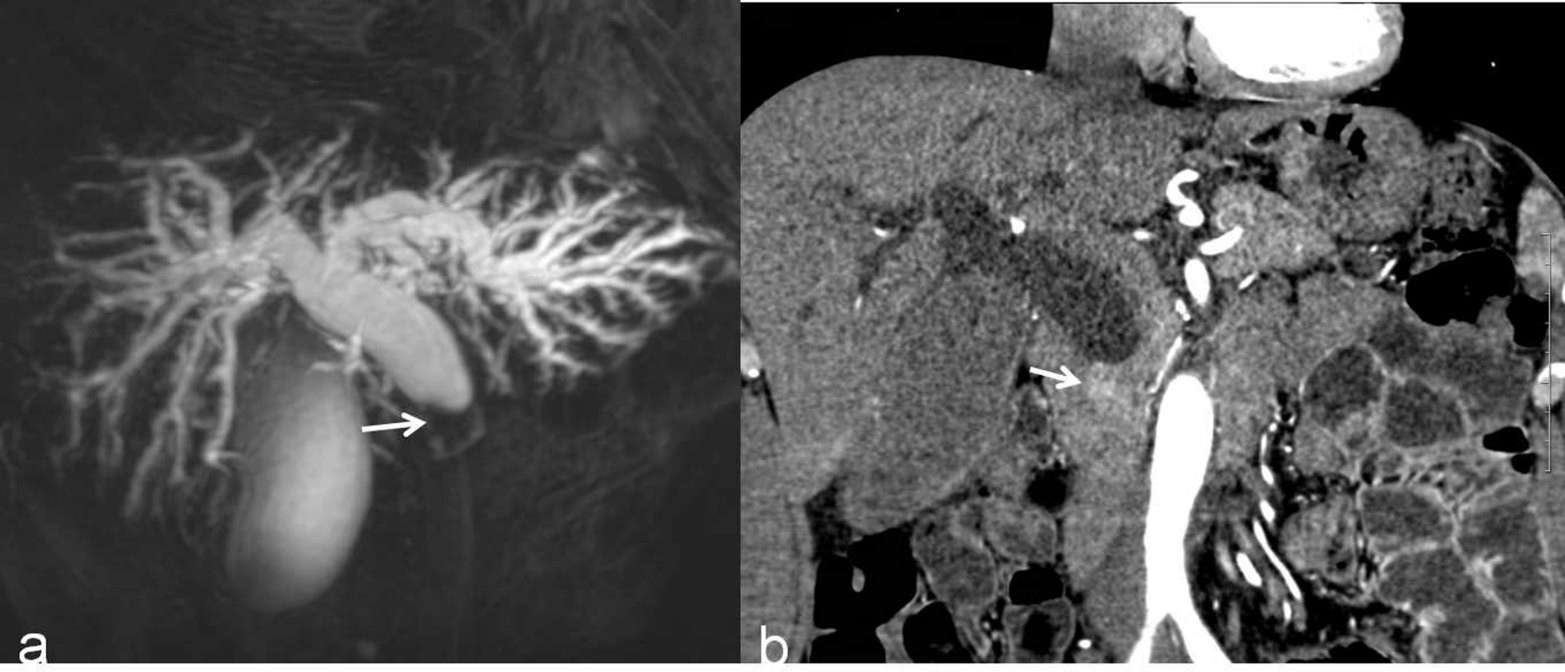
A malignant DBS (score=2) in a 59-year-old man confirmed the lesion as cholangiocarcinoma after surgery. (A) A 3D MRCP image shows abrupt, asymmetrical occlusion with irregular margins (white arrow). The length of the longest segment is 16 mm, the angle of the DBS is 100.4°, and the double duct sign is not present. (B) A coronal CT MPR image in the arterial phase shows the thickened wall with hyperenhancement relative to the liver parenchyma (white arrow).
